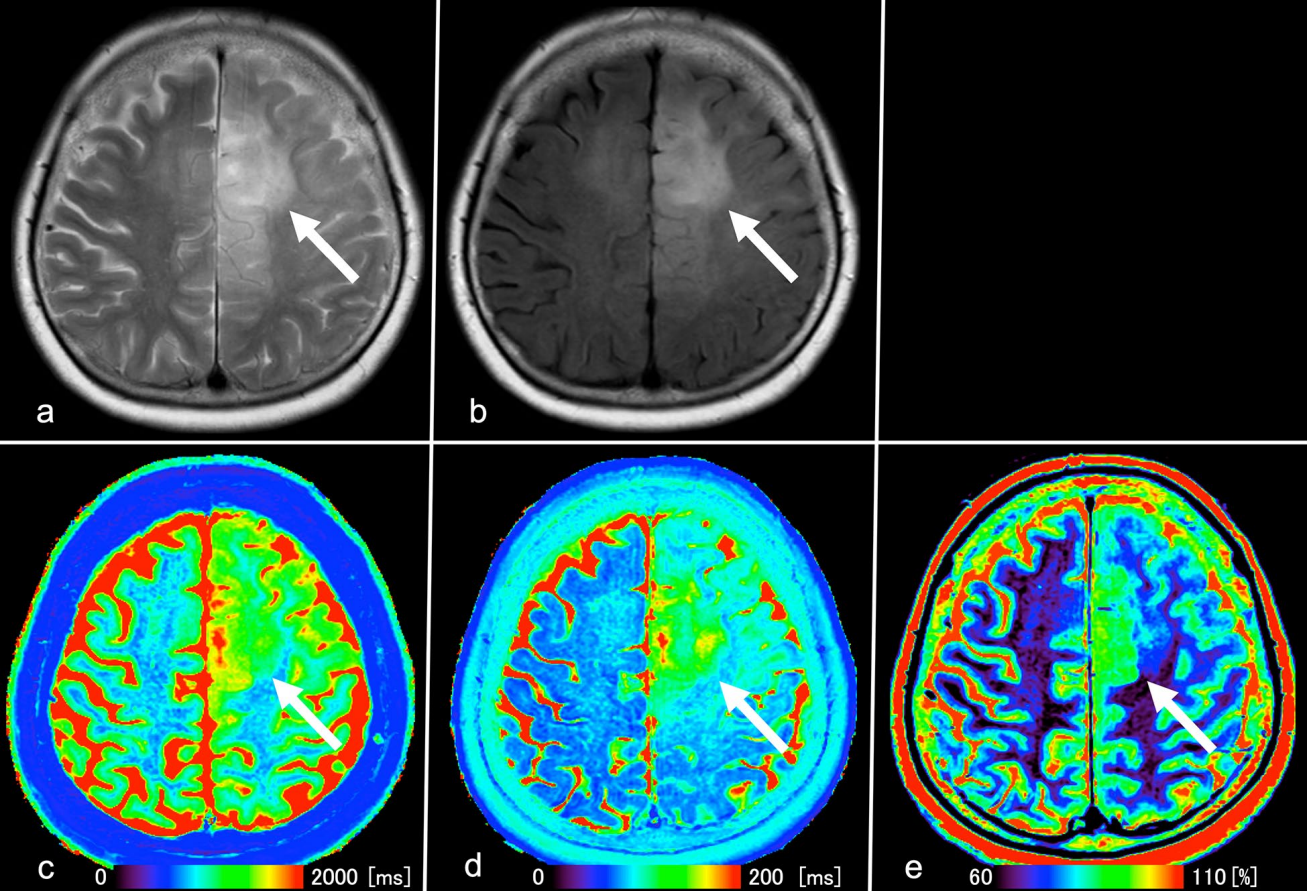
Images from a 63-year-old woman with IDH-mutant and 1p/19q-codeleted oligodendroglioma (WHO grade II). (a) T2WI shows a heterogeneous, poorly circumscribed mass in the left frontoparietal lobes. (b) FLAIR shows no signal suppression in the tumor, indicating no T2-FLAIR mismatch sign (arrow). (c), (d), (e), T1 and T2 relaxation time and proton density (PD) maps derived from SyMRI show mild T1 (1119 ms*) and T2 (103 ms*) relaxation time prolongations and mildly increased PD (76.8%*) (arrows) in the tumor. *Each value is expressed as the mean.
